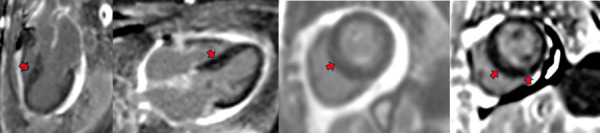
Cardiac magnetic resonance imaging in T1 inversion recovery (IR) sequences, showing late gadolinium enhancement in three-chamber, four-chamber, and short-axis views. Intramyocardial (focal) fibrosis is observed, predominantly in the interventricular septum and inferior wall (red arrows), with a distribution that is atypical for cardiac amyloidosis.
